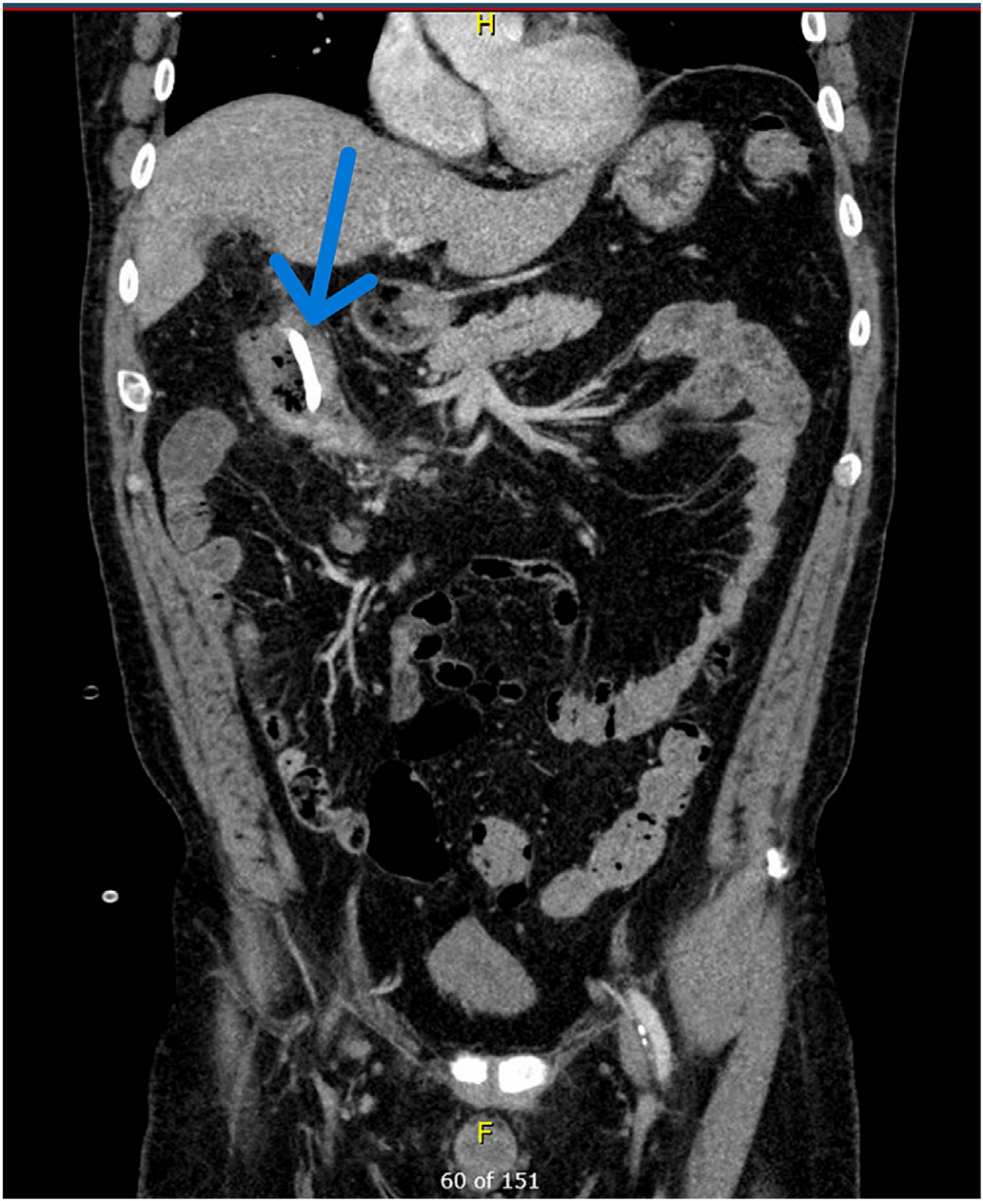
Coronal non-contrast CT abdomen showing abscess catheter within the colonic lumen (blue arrow) approximately 2 weeks after placement. Previously seen abscess is otherwise not well seen on this exam.
